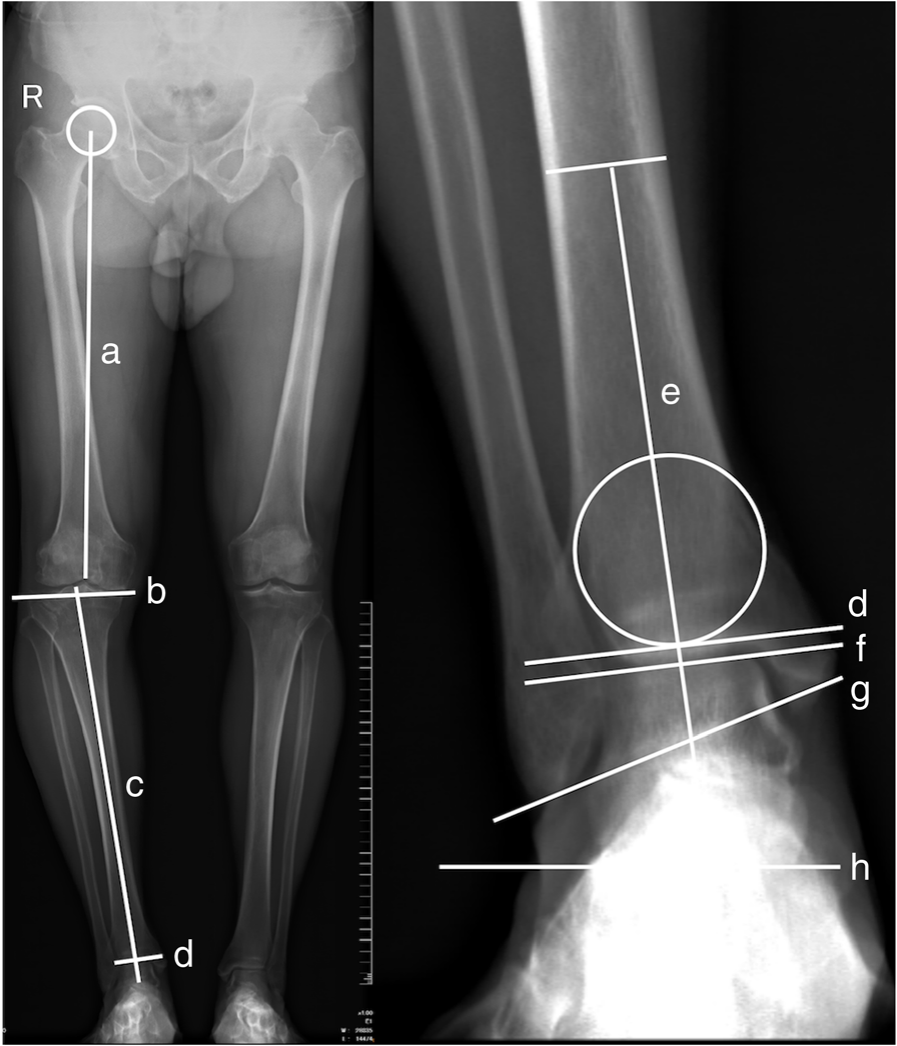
Measurement of HKA and ankle parameters based on full-leg standing anteroposterior radiographs. TTA was defined as the medial angle between “e” and “f.” TAS was defined as the medial angle between “e” and “d.” LDTA was defined as the lateral angle between “c” and “d.” TT was defined as the angle between “d” and “f.” GT was defined as the angles between “f” and “h.” GP was defined as the angle between “d” and “h.” PP was defined as the angles between “b” and “d.” TC was defined as the medial angles between “e” and “g.” Line a was the line from the center of the femoral head to the center of the knee. Line b was the joint surface of the tibial plateau. Line c was the line from the center of the tibial plateau to the center of ankle. Line d was the distal tibial plafond. Line e was the anatomical axis of the tibia. Line f was the upper joint surface of the talus. Line g was the line from medial malleolus to lateral malleolus. Line h was the ground surface
